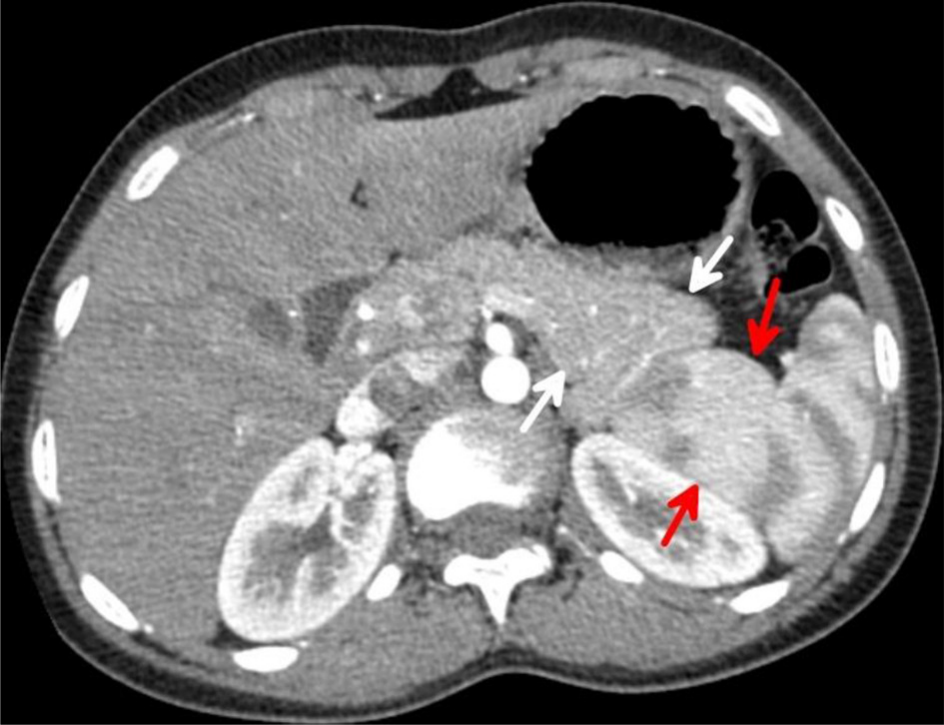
Transverse contrast-enhanced CT images acquired during the arterial phase through the mid-spleen demonstrates the medial internal lobe of the spleen (red arrows) insinuated between the compressed tail of the pancreas (white arrows) and the left kidney. The mottled (zebra lines) enhancement pattern of the spleen during the arterial phase distinguishes the medial (internal) lobe of the spleen and differentiates it from the adjacent tail of the pancreas and upper left kidney.
